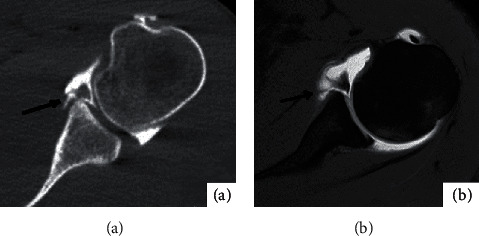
Male, 29 years old, with recurrent anterior dislocation of left shoulder for half a year. (a) shows the bone window image in the transverse axis of the left shoulder CTA, and (b) shows the T1WI-FS image in the transverse axis of the left shoulder MRA. All the black arrows show local nonunion of the anterior lower lip and contrast agent entering the fissure.
